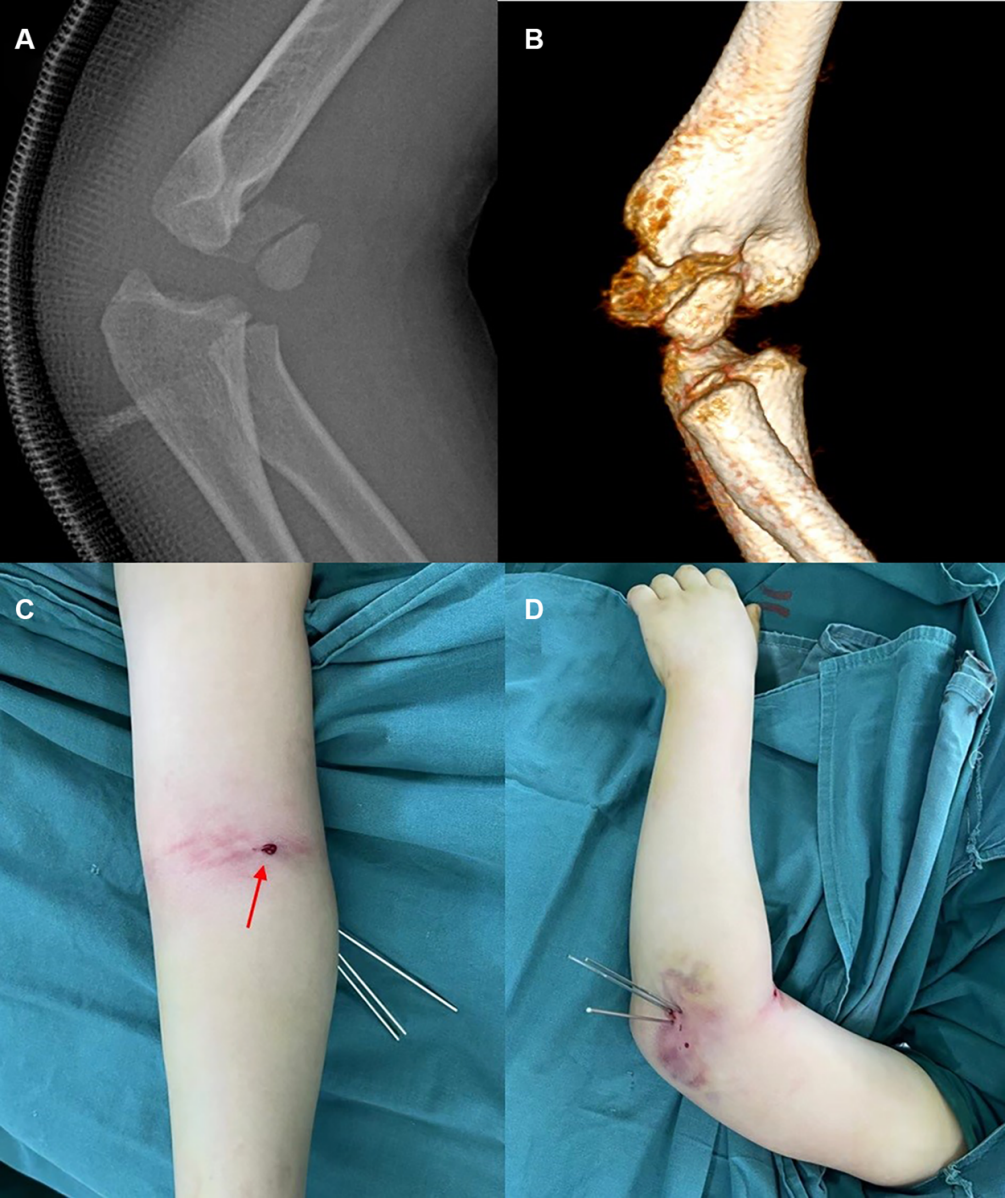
Typical x-ray of Type II degree II fractures. Lateral (A) radiograph and 3D CT (B) radiograph showing a severe fracture with rotation and antero-proximal displacement of the distal fragment. The fracture is classified as a Type II degree II (unstable) fracture. Aspect (C) of leverage reduction from the anterolateral side (red arrow). Aspect (D) of the elbow after K-wire fixation.
